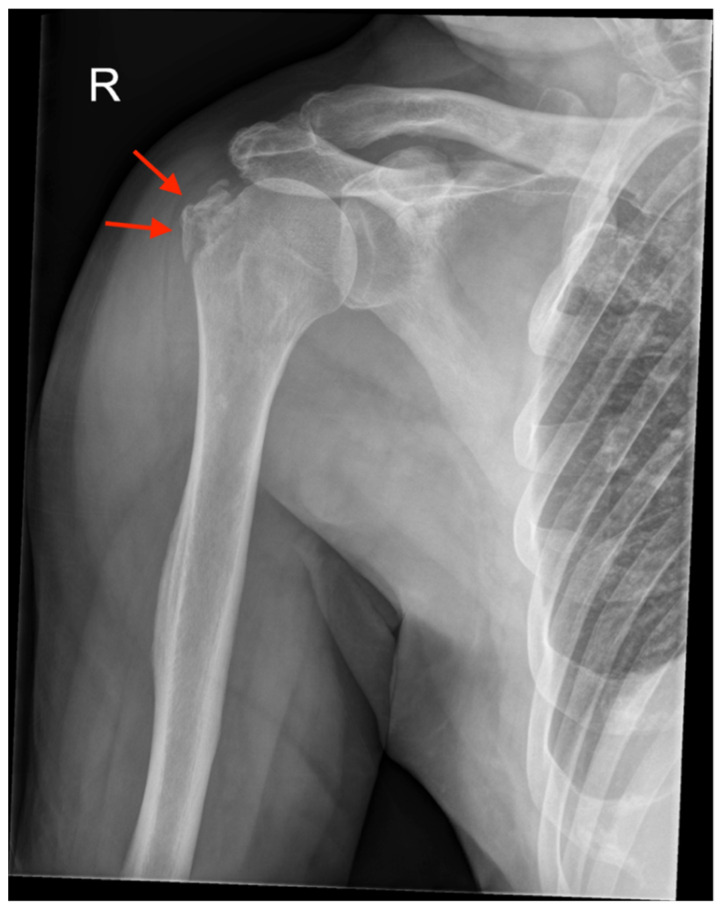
Plain radiography of the right shoulder with inferior dislocation after reduction: the greater tuberosity is fractured and nearly undisplaced after reduction (red arrows).
